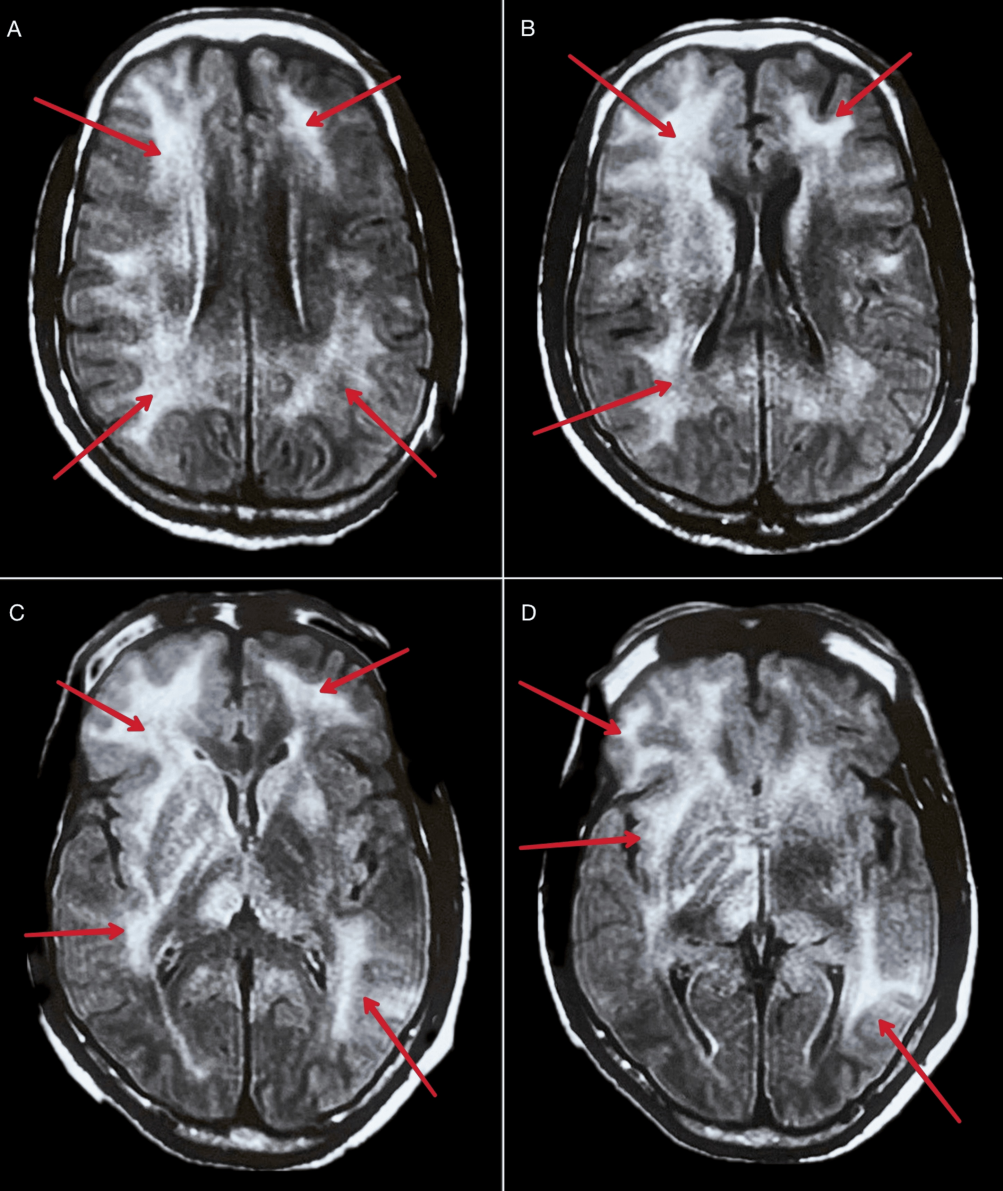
Brain MRI images, marked with red arrows, demonstrate diffuse white matter hyperintensities across multiple regions on FLAIR sequences. Panels A and B show hyperintensities in the bilateral frontal regions; panels C and D highlight similar findings in the temporal regions; and panels B, C, and D reveal the involvement of the parietal periventricular regions.
